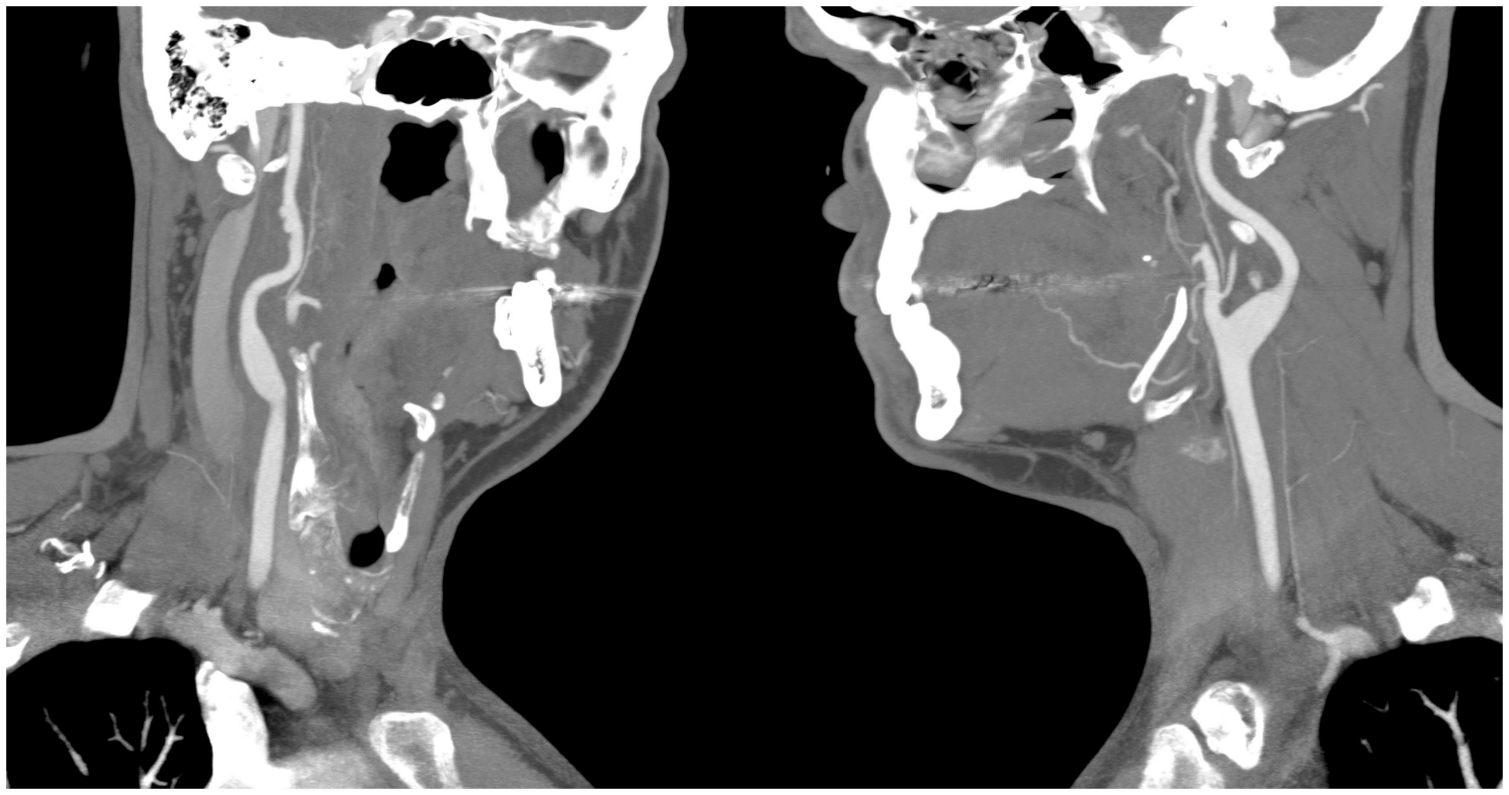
CT angiography showing bilateral pseudoaneurysms with the (left panel) showing the patient's right internal carotid artery, and the (right panel) showing the patients left internal carotid artery.
